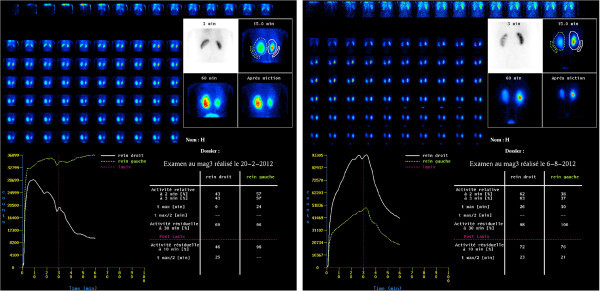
99 mTc-labelled mercaptoacetyltriglycine (MAG-3) diuretic renal scintigraphy. On the left: before surgery and on the right: after surgery.
